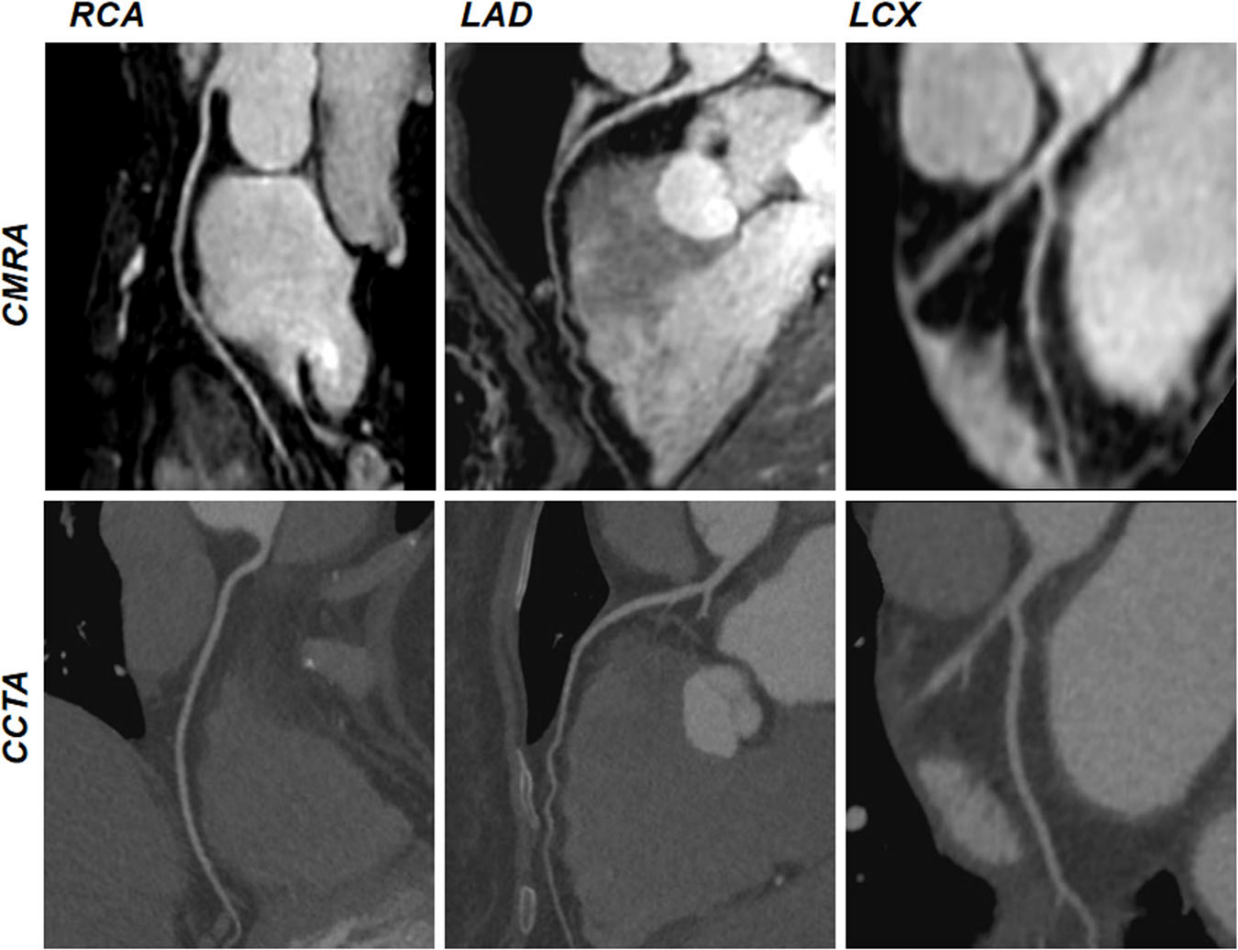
Non-contrast DL-CS mDIXON coronary MRA images of a 60-year-old female patient with normal coronary arteries. coronary MRA images are depicted in the top row, displaying the right coronary artery, LAD artery, and left circumflex. The corresponding reformatted images obtained with contrast-enhanced CCTA are shown in the bottom row. CMRA, coronary magnetic resonance angiography; CCTA, coronary computed tomography angiography
